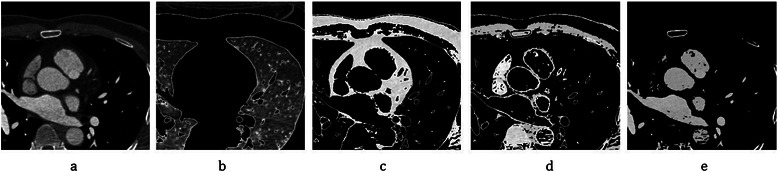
CTA data categorization based on Hounsfield Unit (*HU*). (a) A selected CTA slice as an input. (b) Region 1 containing pulmonary tissues. (c) Region 2 including the diaphragm and pericardium. (d) Region 3 including uncontrasted coronary arteries, myocardium, and hepatica tissues. (e) Region 4 including contrasted coronary arteries, ventricles, bone, aorta, pulmonary vessels.
